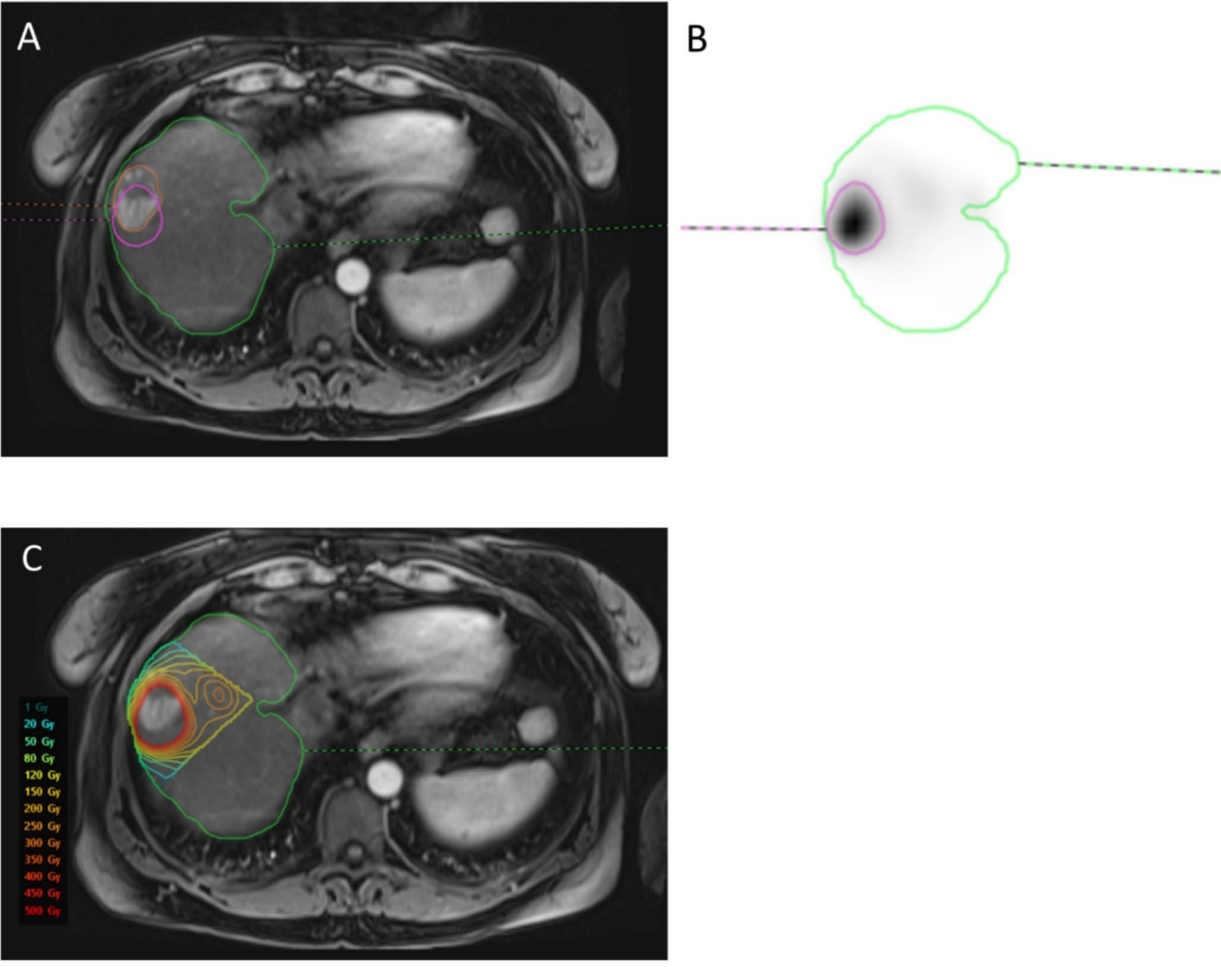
The hypervascular lesion in segment 8 (A) does not align with the high focal [^99m^Tc]TcMAA accumulation on SPECT (B; purple color), favoring the [^99m^Tc]TcMAA threshold segmentation method to capture the most accurate tumor absorbed dose (C)
